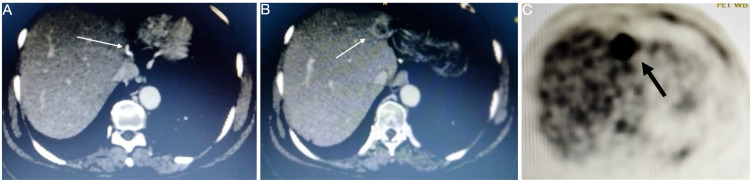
(A) Follow-up CT after left lateral segmentectomy showing surgical staples (line arrow) applied to the left hepatic vein, (B) Recurrence of the lesion in the segment IVa (line arrow), and (C) Corresponding PET image showing FDG uptake in the lesion (black arrow). PET: Positron emission tomography; FDG: Fluorodeoxyglucose.
